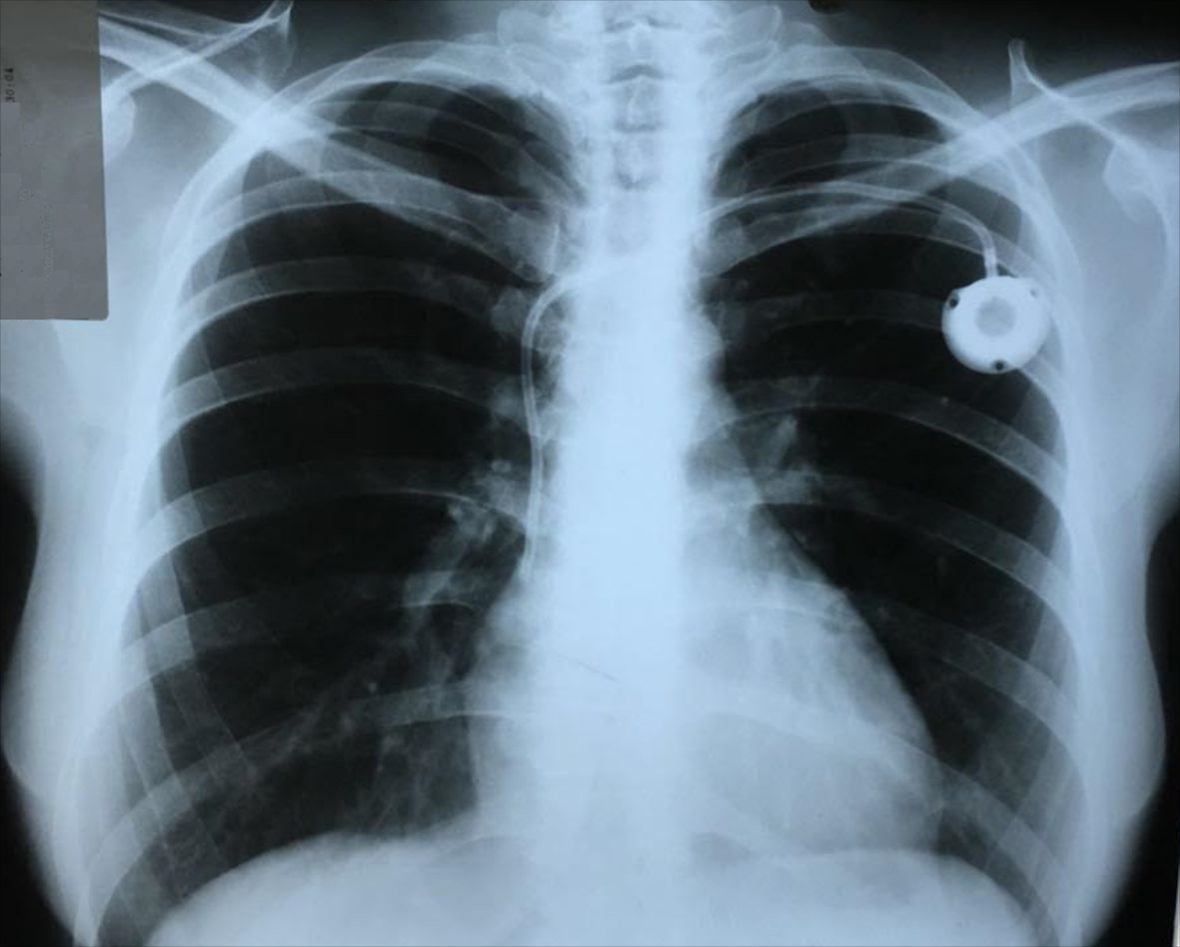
Anteroposterior chest radiograph taken in the immediate postoperative period, showing unremarkable positioning of the catheter.
